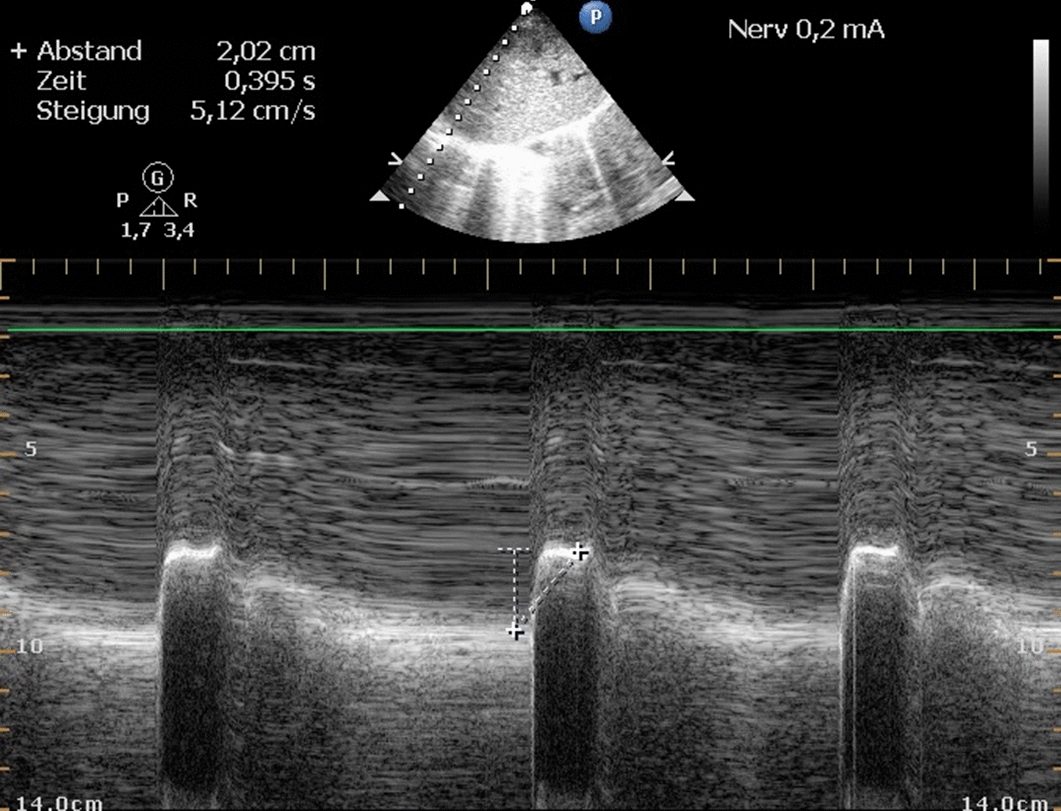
Measurement of diaphragm movement by ultrasound (M-mode) under stimulation of the phrenic nerve with 0.2 mA (three measurements were made for data recording, from which the mean value was calculated)
